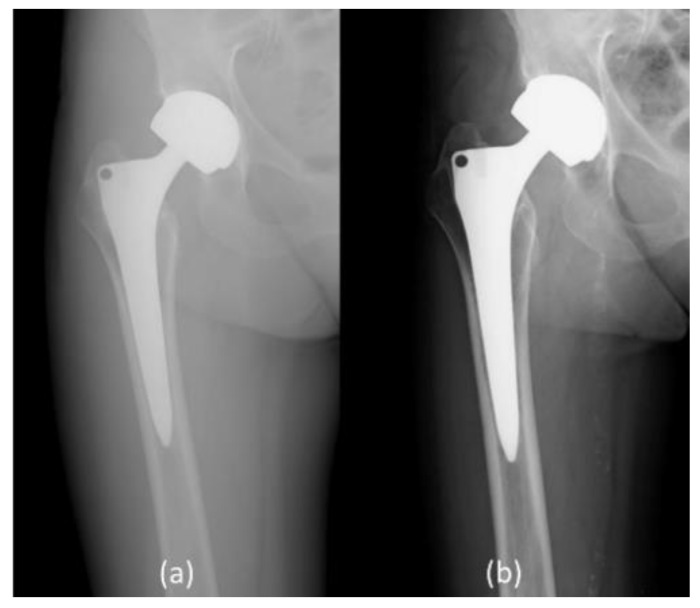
F.A., 81-year-old female, Metal-on-Metal Total Hip arthroplasty for primary arthrosis, right hip X-ray. (a) Post-operative follow-up, one month (b) Post-operative follow-up, 7 years (24 months after recruitment).
